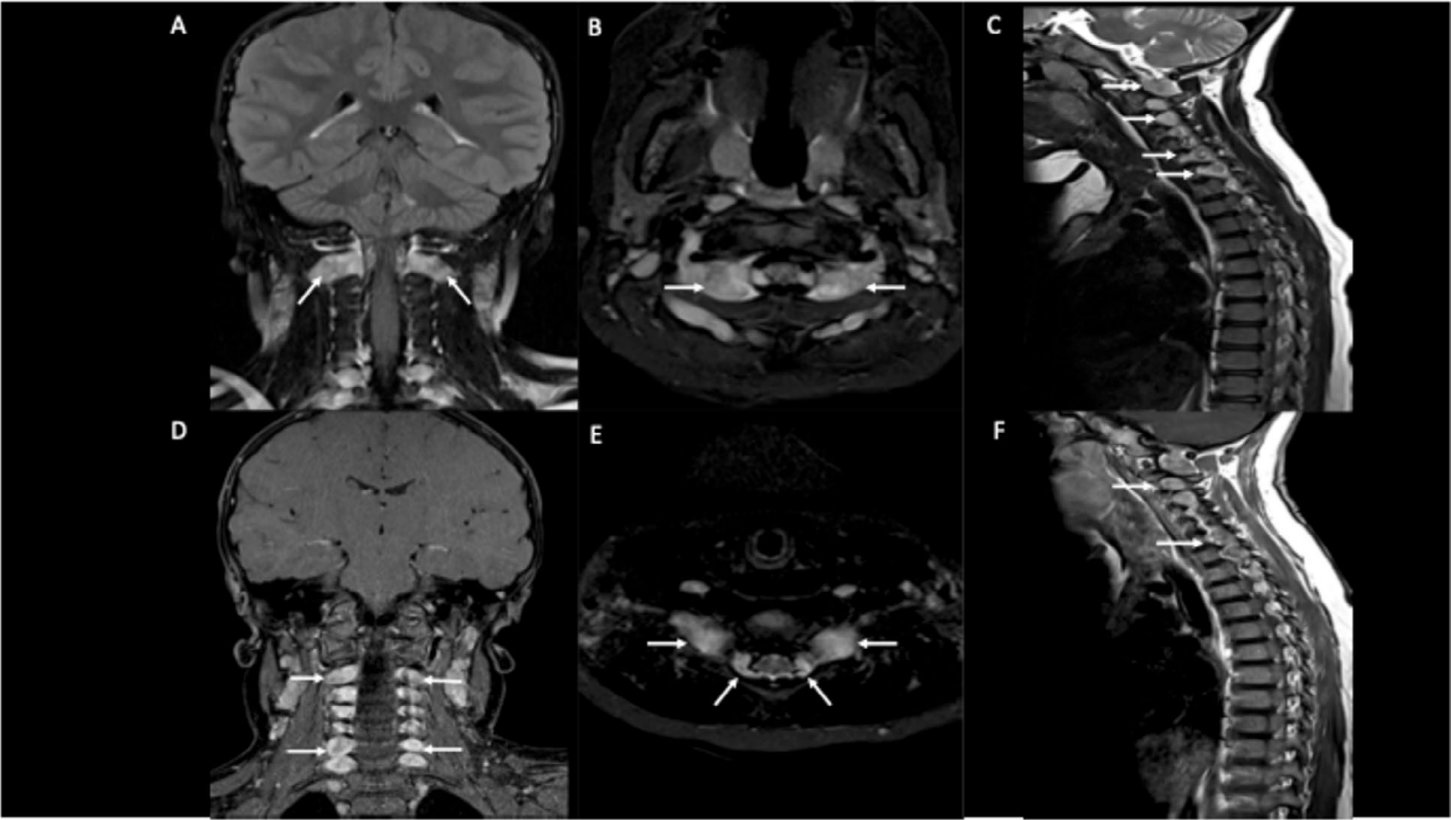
MRI of the brain and spine showing multiple masses in the bilateral neuroforamina of the cervical spine, in keeping with neurofibromas (arrowed a–f), which enhance after contrast administration (d, f). Sagittal imaging of the spine at 6 years on T2 imaging (c) and on contrast‐enhanced T1imaging (f).
